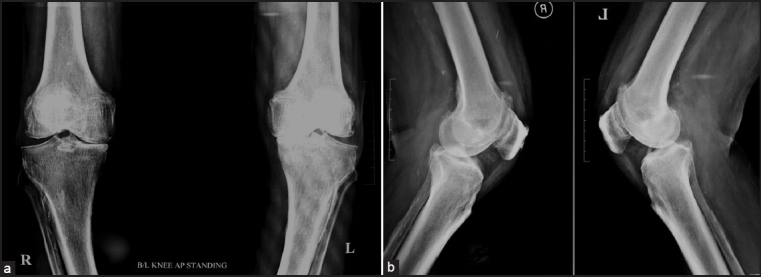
Preoperative anteroposterior (a) and lateral (b) radiographs of both knee of 81-year old patient showing evidence of tri-compartment arthritis
